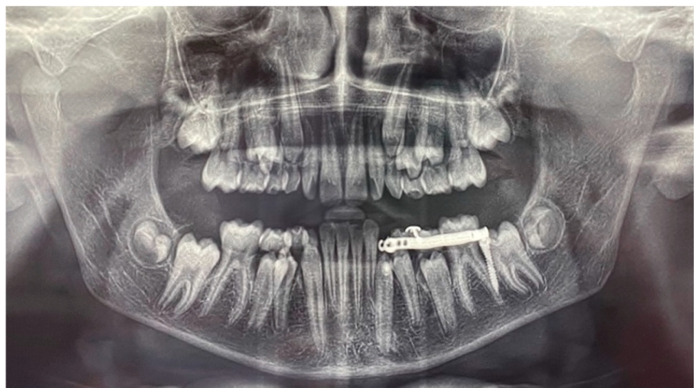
Panoramic radiograph at the end of the orthodontic traction with the customized skeletal anchorage device.
